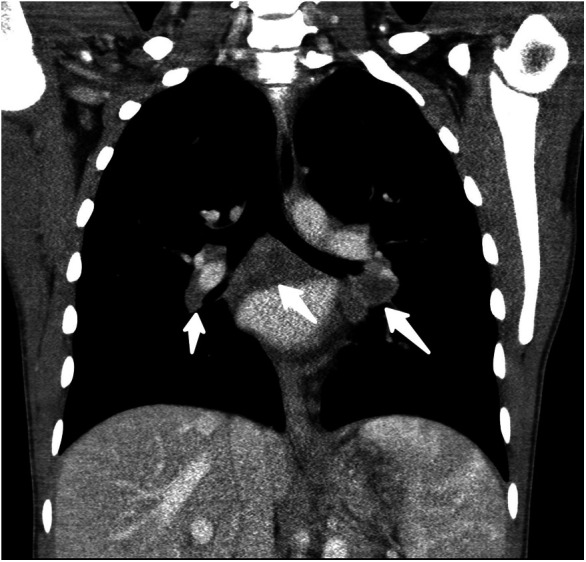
Coronal reformatted, soft-tissue window, CT image through the mediastinum with low attenuation, noncalcified, bihilar, and subcarinal adenopathy (white arrows).
